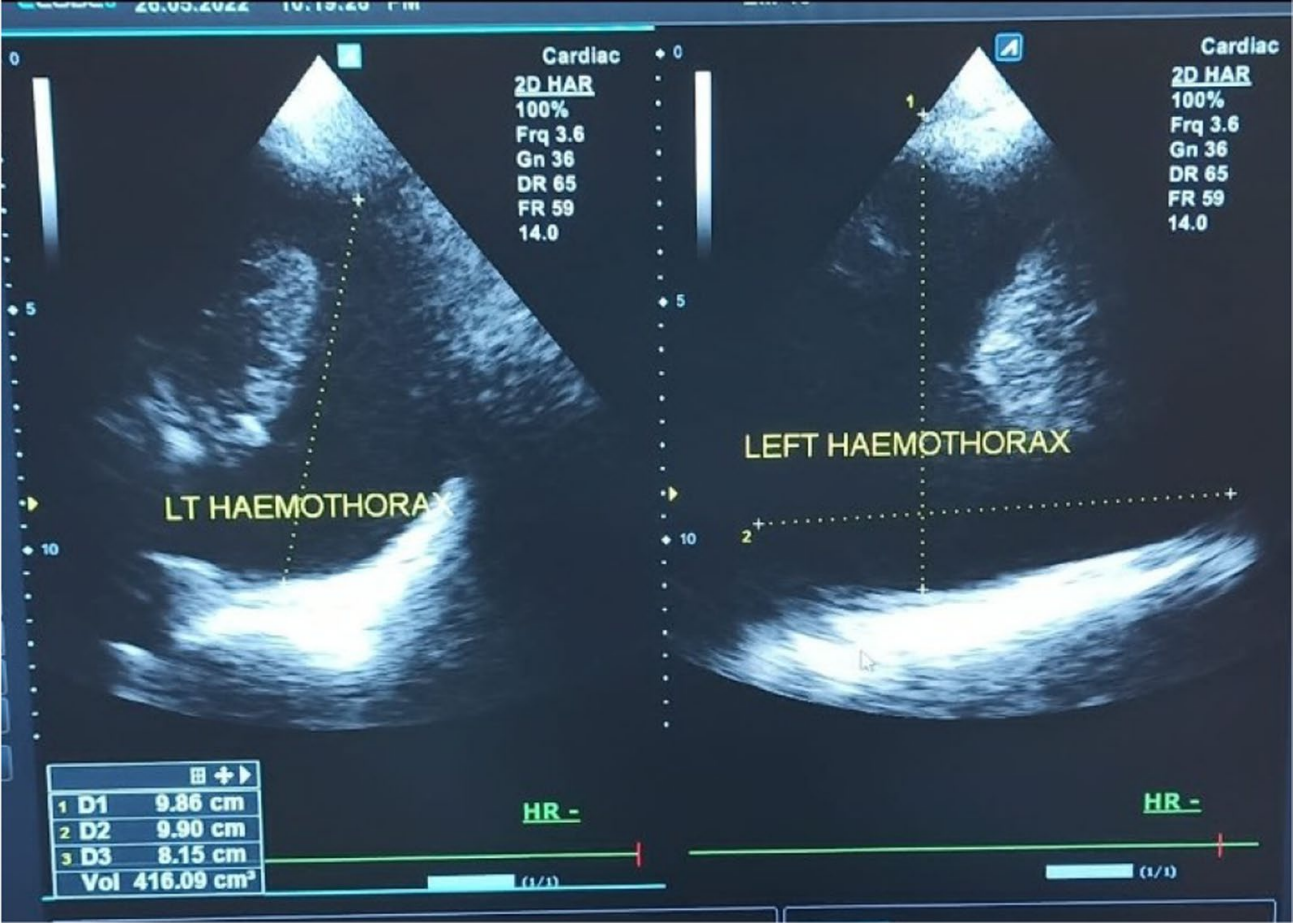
Shows a sonographic image of left haemothorax in a 30 year old male participant
